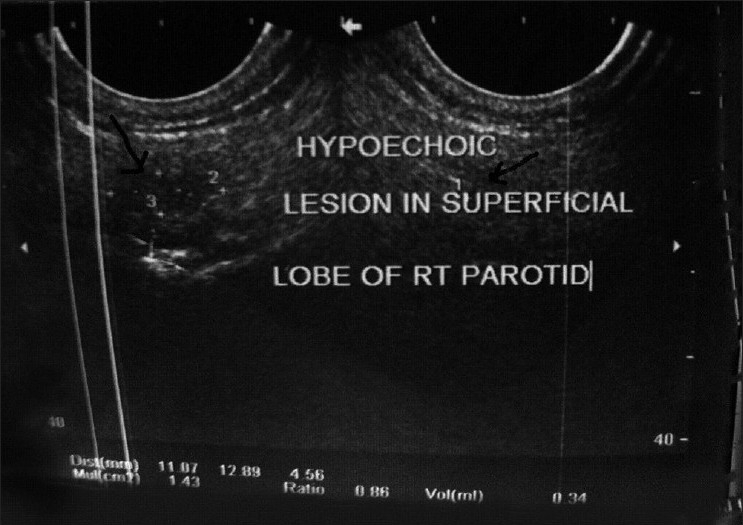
High-resolution sonography of the parotid region revealed a low level echo complex mass in superficial lobe of right parotid (size 32 × 17 mm) with no calcification or cystic degeneration, suggestive of inflammatory mass.
